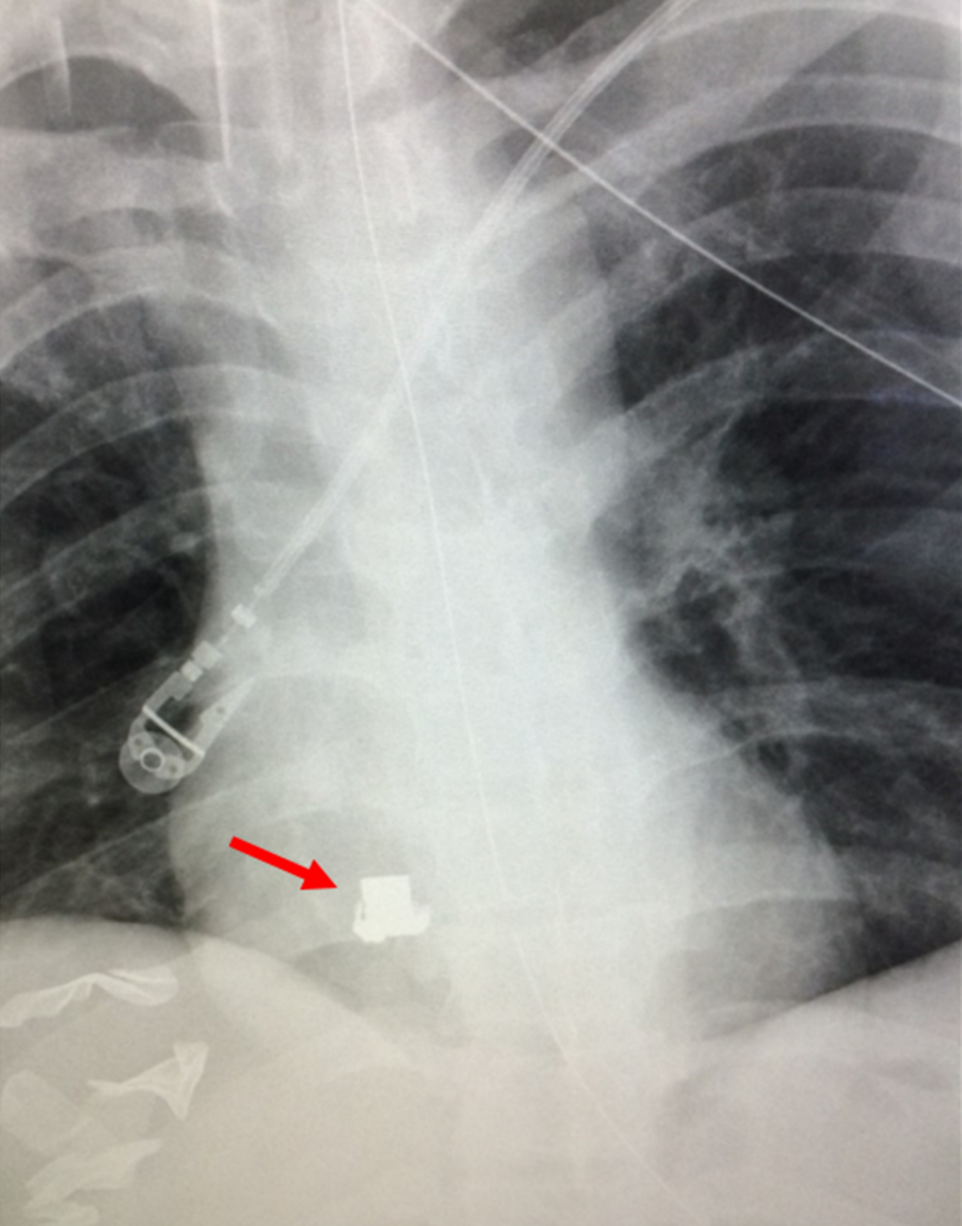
Post-Surgical Chest Radiograph A post-surgical chest radiograph is shown after surgical clamping of the patient's inferior vena cava. A radiopaque bullet is shown overlying the right heart (red arrow).
